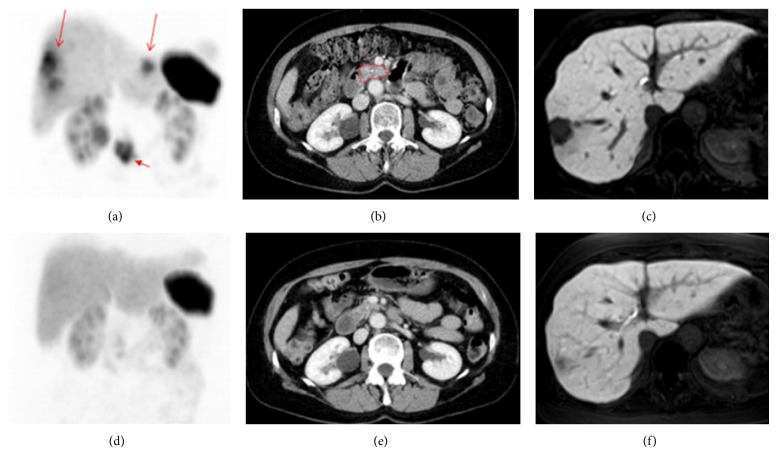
Patient 5 before therapy ((a)–(c)) and after three cycles of ^213^Bi-DOTATOC ((d)–(f)) to a dose of 4 GBq. (a) Beta-resistant residuals in the liver (long arrows) and primary tumor (short arrow) are present in the ^68^Ga-DOTATOC PET maximum intensity projection image. (b) Contrast enhanced CT image with the primary tumor outlined in red. (c) In the MR image with hepatocyte-specific contrast medium, the liver metastases appear as black cavities against the enhancing normal liver parenchyma. ((d)–(f)) After three cycles of ^213^Bi-DOTATOC to a dose of 4 GBq, the lesions have diminished on the PET image (d) and CT image (e). Also on the MR image (f), the residual lesion has almost disappeared as shown by the growth of normal hepatocytes demonstrated by the uptake of the hepatocyte-specific contrast medium [36].
